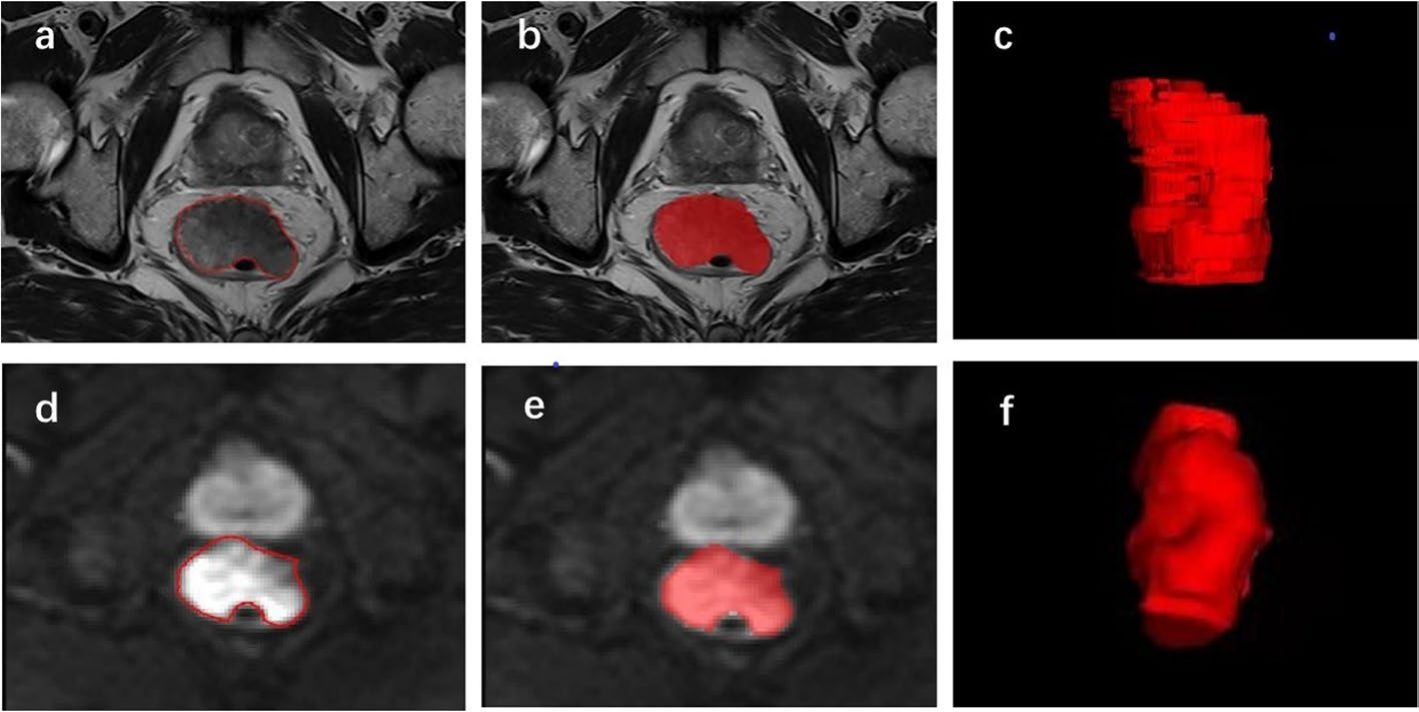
Example of segmentation. Images from a 73-year-old man with rectal adenocarcinoma and pathologically proven lymphovascular invasion. a, b Delineation of the tumor on T2-weighted images. d, e Delineation of the tumor on diffusion-weighted images. c, f Three-dimensional rendering of segmentation of tumor
